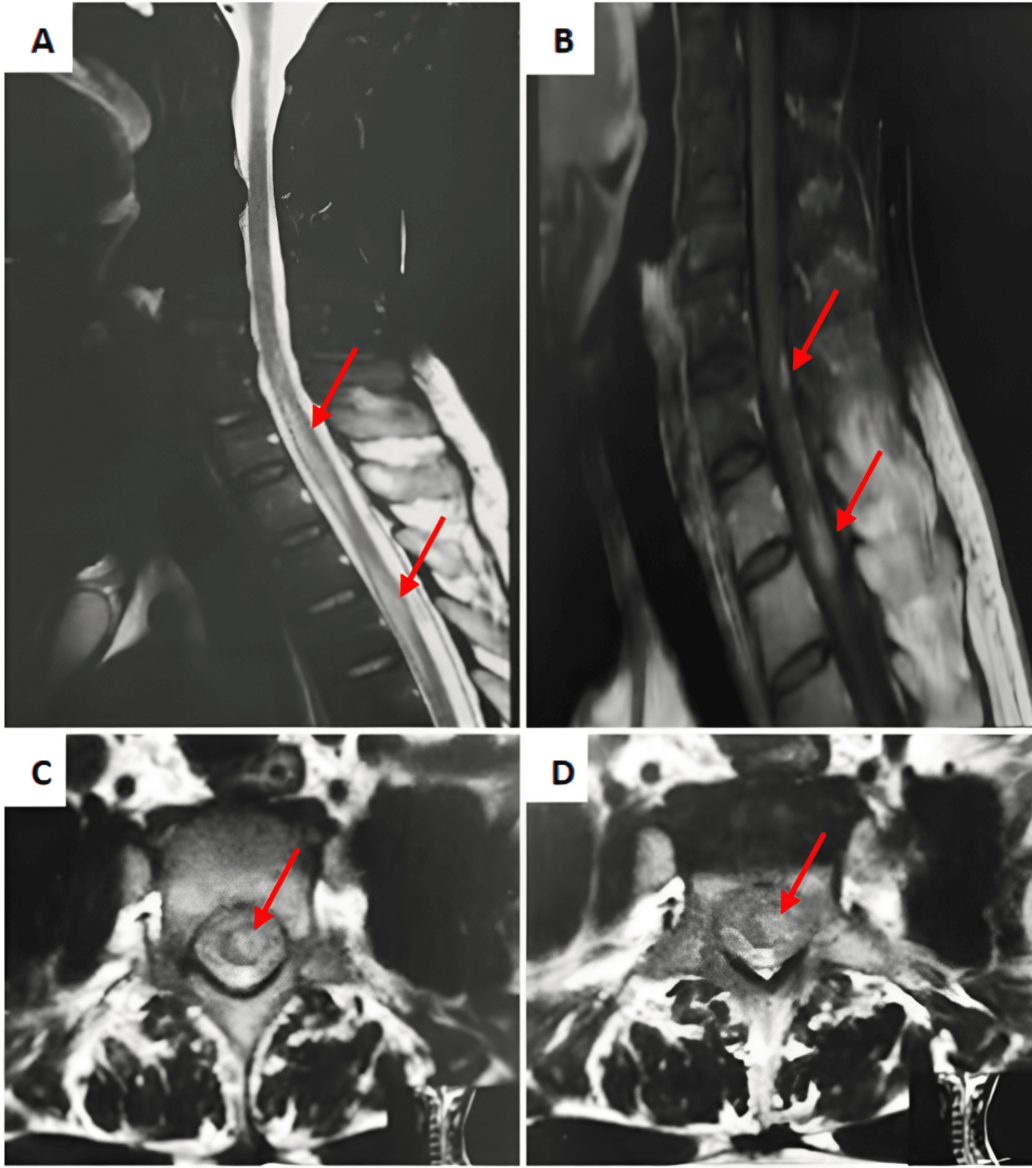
MRI of the cervical and thoracic spine demonstrating intramedullary spinal cord metastases (red arrows in images A, B, C, and D). (A) Sagittal T2-weighted MRI showing a hyperintense lesion with surrounding edema. (B) Sagittal post-contrast T1-weighted MRI showing a peripheral thin region of increased enhancement (rim sign) associated with an ill-defined enhancement extending below the lesion. (C-D) Axial T2-weighted MRI.
